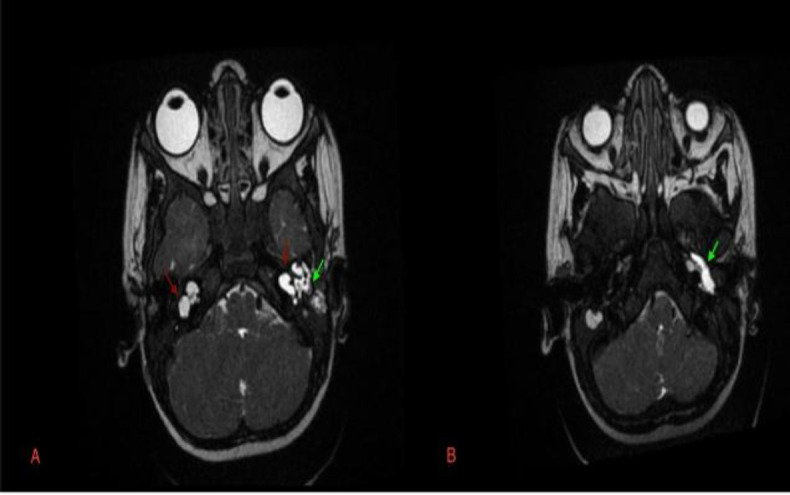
T2 Magnetic resonance images axial section showing bilateral inner ear malformation (red arrows) with CSF leaking to middle ear on left side (B) CSF seems extending from middle ear to eustachian tube on left side (green arrows)
